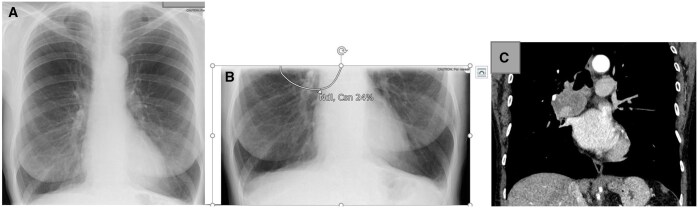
A 71-year-old female ex-smoker with increasing breathlessness underwent a CXR (A). Due to the lung bases being omitted from this image, a second lung bases X-ray image was acquired (B). Both images (A and B) were reported as NAD, with the AI software being NAD for image (A) but suspicious of a nodule on the subsequent image (B) only. Ten months later, the patient returns for a CT scan (C) due to haemoptysis, demonstrated a right hilar mass later confirmed to be stage IV LC. AI = artificial intelligence; CXR = chest radiograph; LC = lung cancer; NAD = no abnormility detected.
